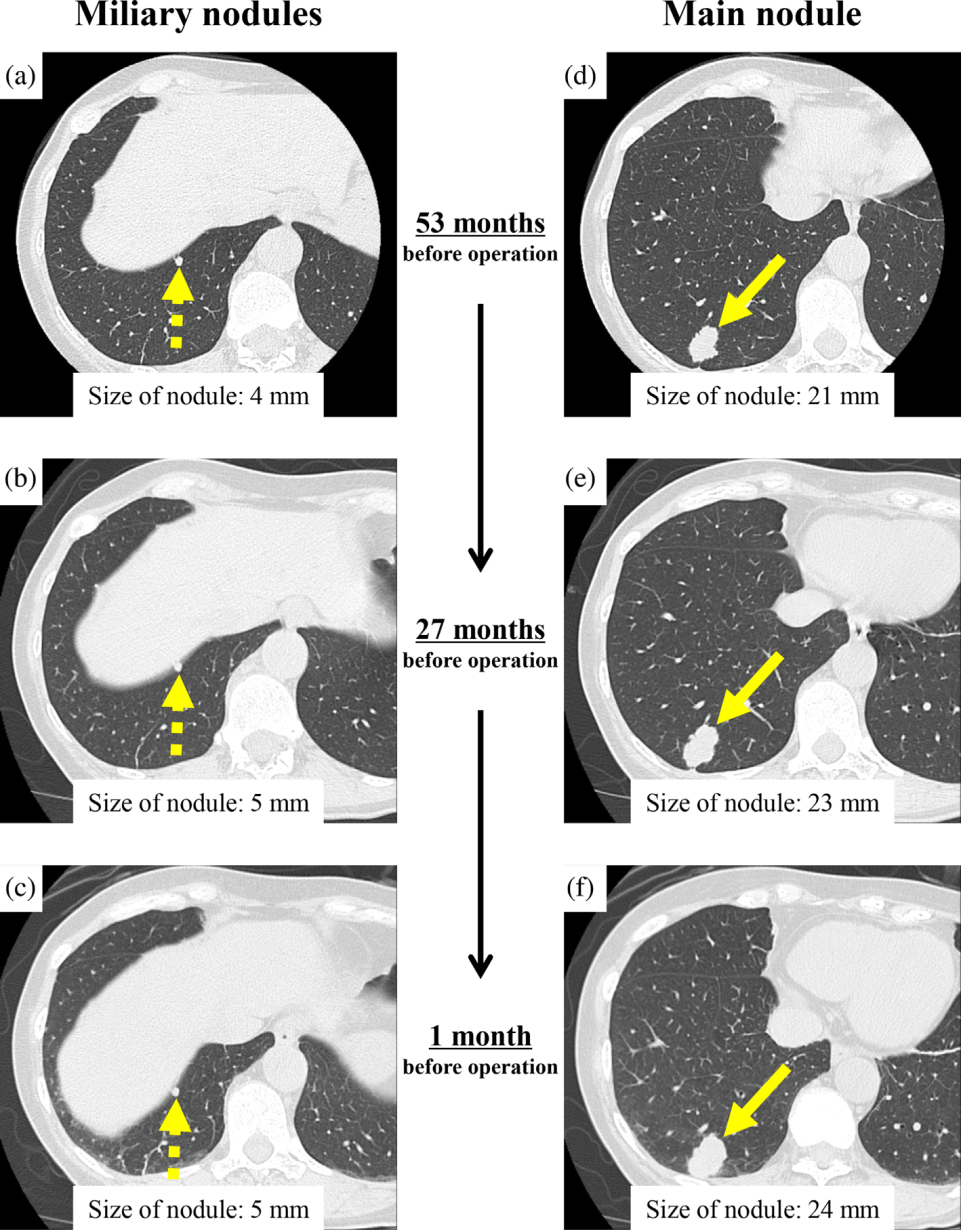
Computed tomography (CT) scan findings of lung nodules. (a–c) Multiple miliary nodules followed up for 17 years were found (dashed arrow). (d–f) A main nodule showing slow enlargement was detected in the right lower lobe (solid arrow). Chronological CT scans at 53 months (a, d), 27 months (b, e) and 1 month (c, f) before the operation demonstrate miliary nodules (a–c) remained stable, while the main nodule (d–f) was slowly enlarging.
